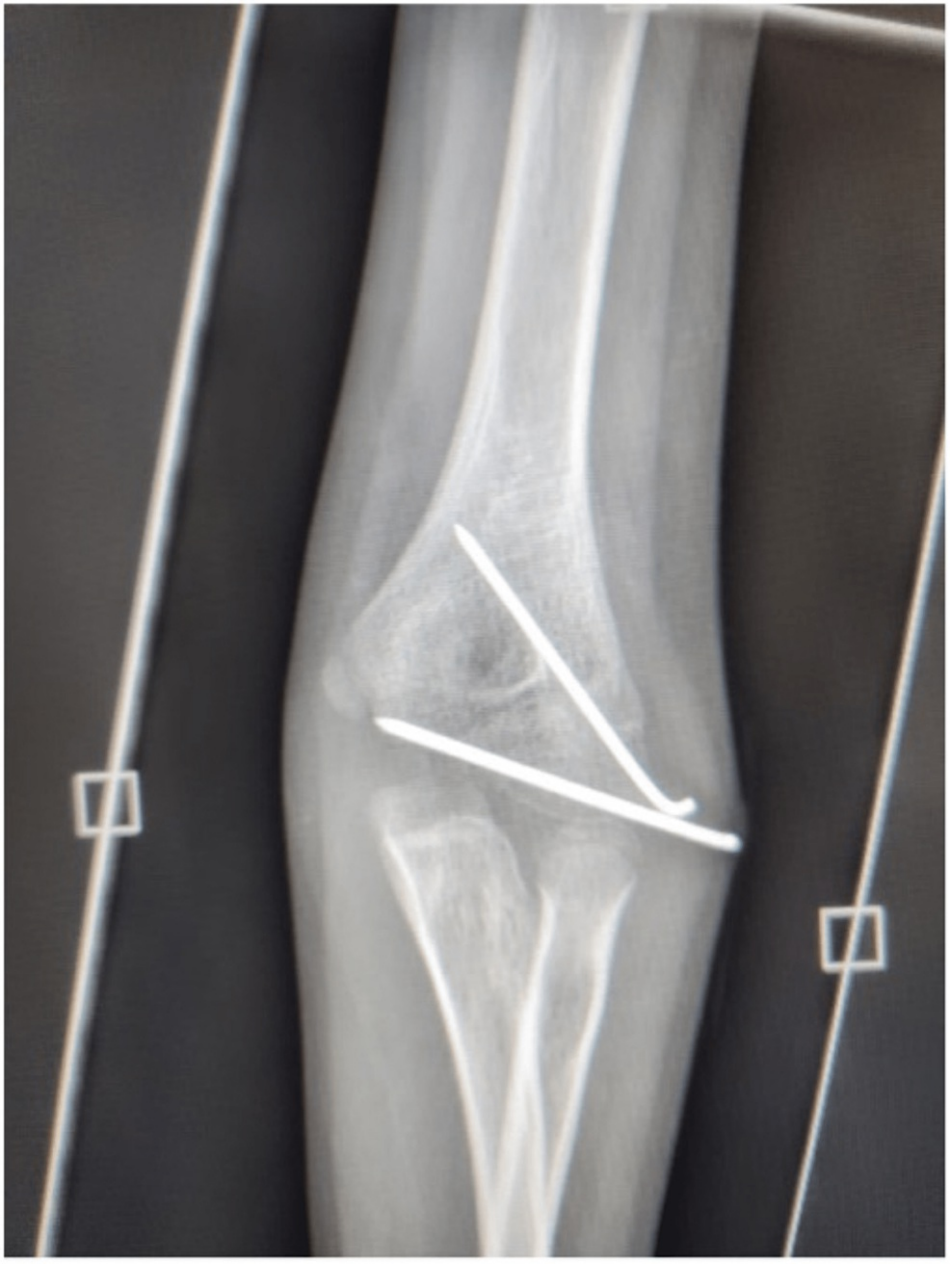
During the six-week follow-up appointment, X-rays were taken, which showed that the lateral humeral condyle fracture had achieved solid union
